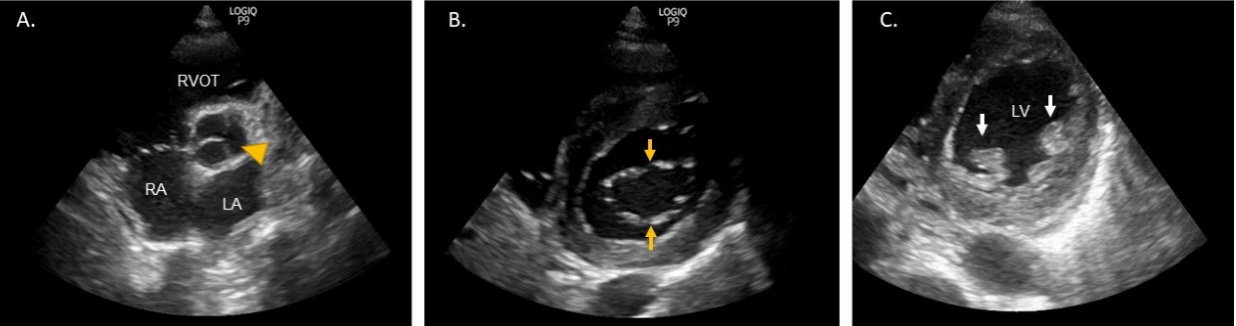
Parasternal short axis (PSAX) view at the level of A) aortic valve [arrowhead], B) mitral valve [arrows point to the valve leaflets], and C) papillary muscle/mid-ventricle [arrows point to papillary muscles]. RA: right atrium, LA: left atrium, RVOT: right ventricular outflow tract, LV: left ventricle.
